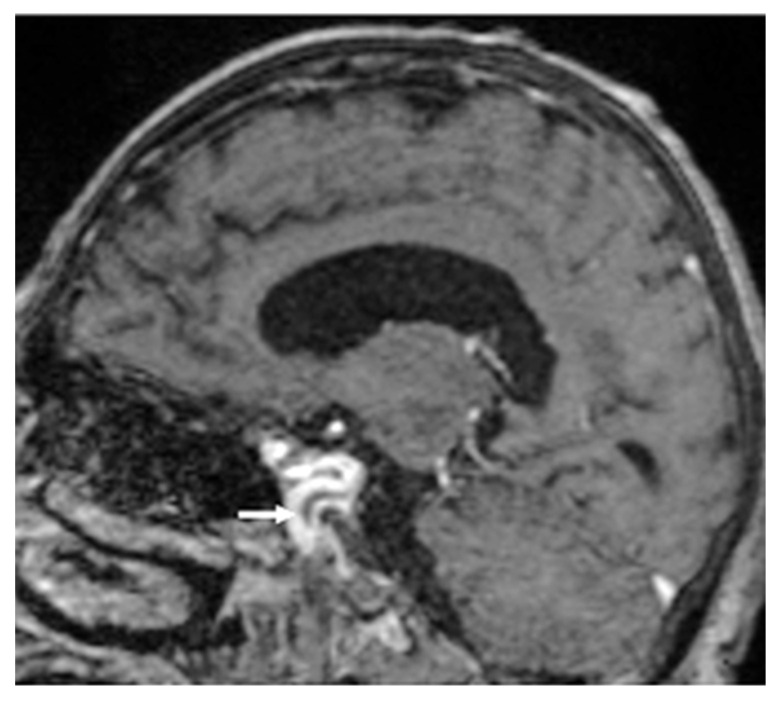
Sagittal T1-weighted Spin Echo Sequence in MRI showing heterogenous filling defect in the left cavernous sinus, suggestive for thrombosis marked with the white arrow.
